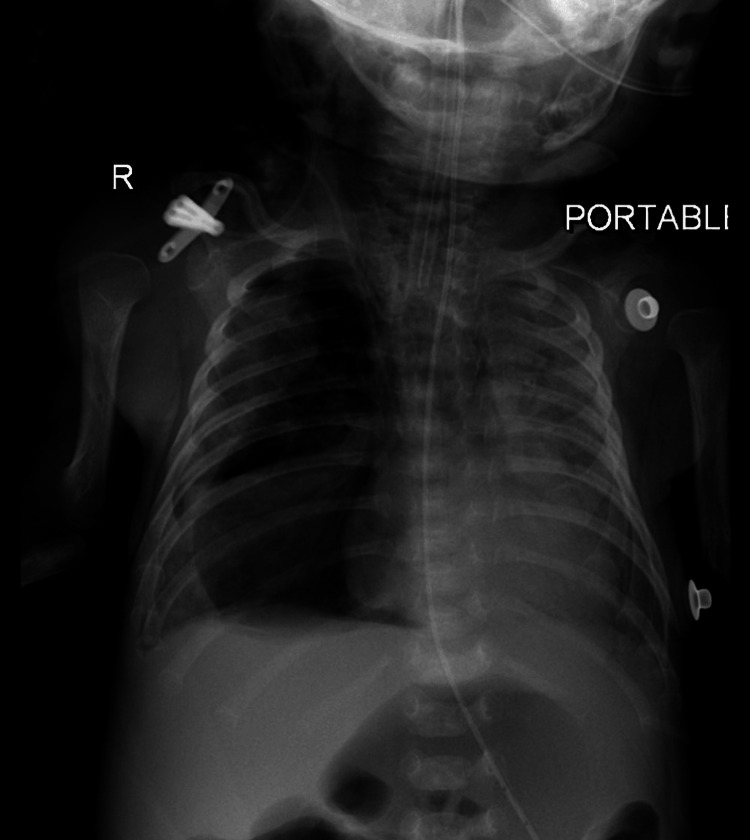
Chest X-ray (anteroposterior view) done two weeks after admission showing infiltrative lung disease and a progressive course of left lung inhomogeneous opacities.
